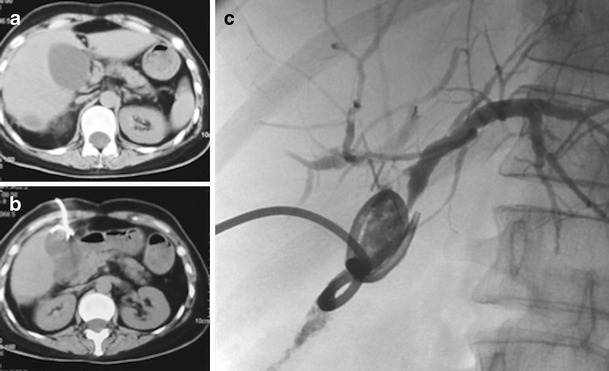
a Laceration and obstruction of common hepatic duct post laparoscopic cholecystectomy that led to biloma formation. b Percutaneous CT-guided drainage of the biloma. c Cholangiographic picture that confirms the communication of the collection with the biliary system. Surgical repair of the bile ducts followed
